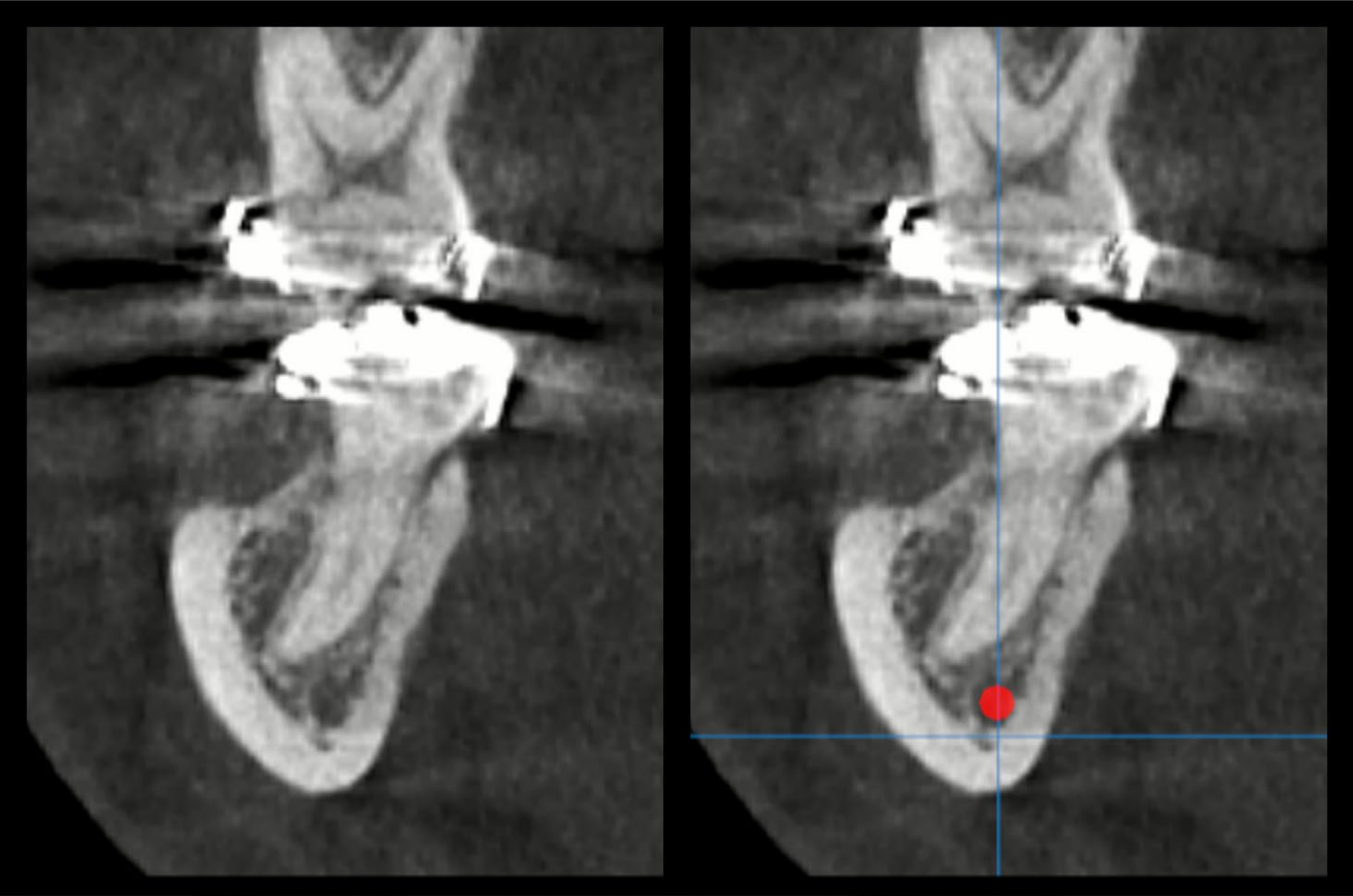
Cross‐sectional cone‐beam computed tomography image of the posterior mandibular region, highlighting the spatial relationship between the root apex of a molar and the mandibular canal (solid red circle).
